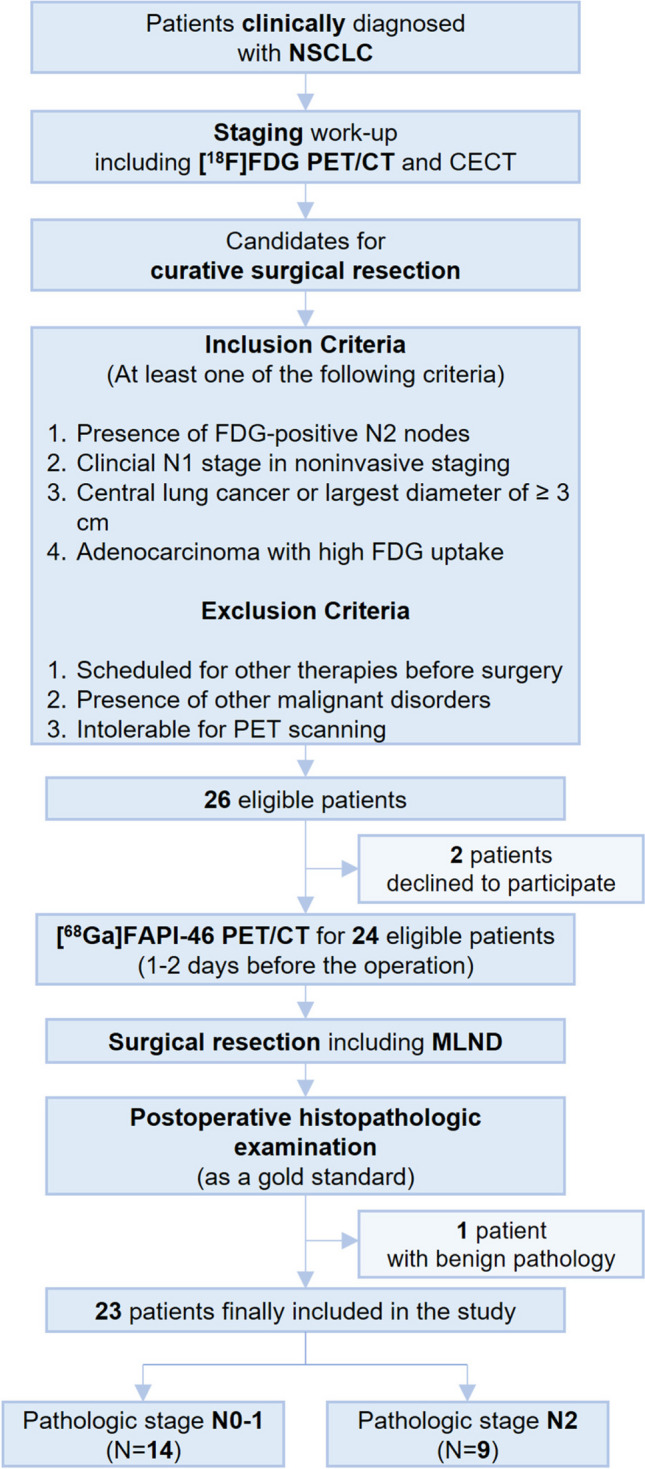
Overall study flow. *FAPI* fibroblast activation protein inhibitor; *CECT* contrast-enhanced computed tomography; *MLND* mediastinal lymph node dissection
